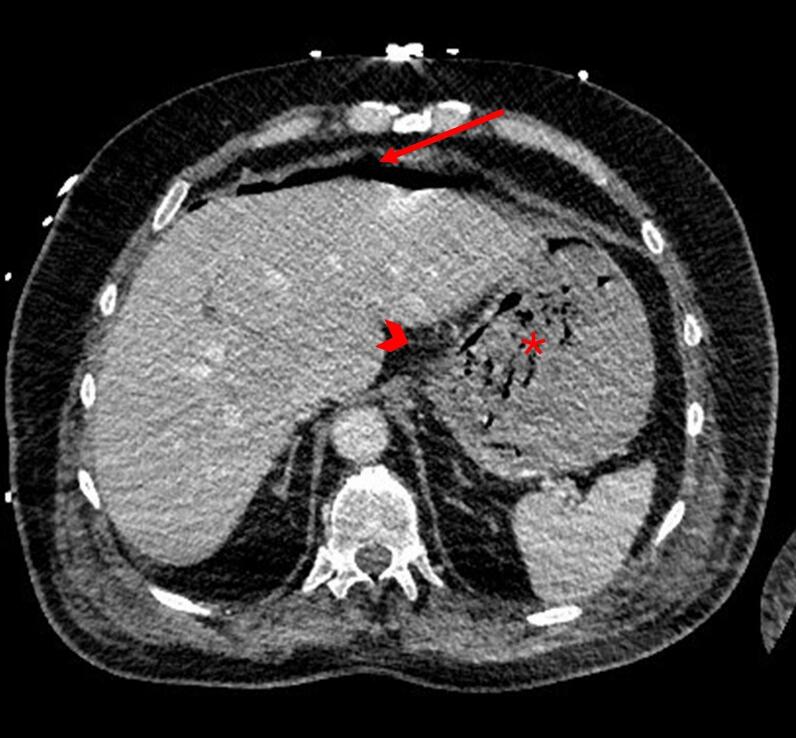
CT scan showing supra-mesocolic pneumoperitoneum (arrow), perigastric fat stranding (arrowhead) and intragastric blood (star).
